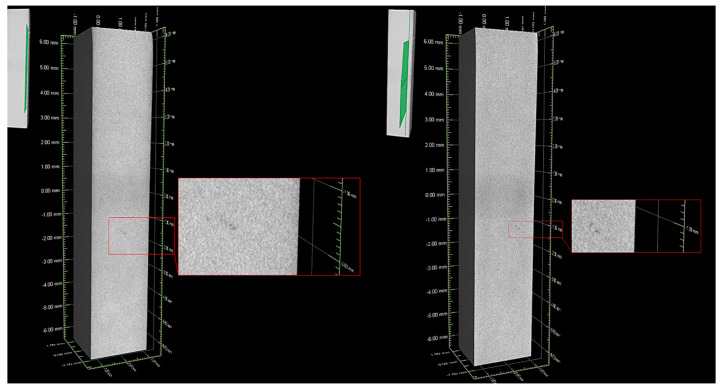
Computed tomography images of the remelted Inconel 740 used for the chemical composition modification.
